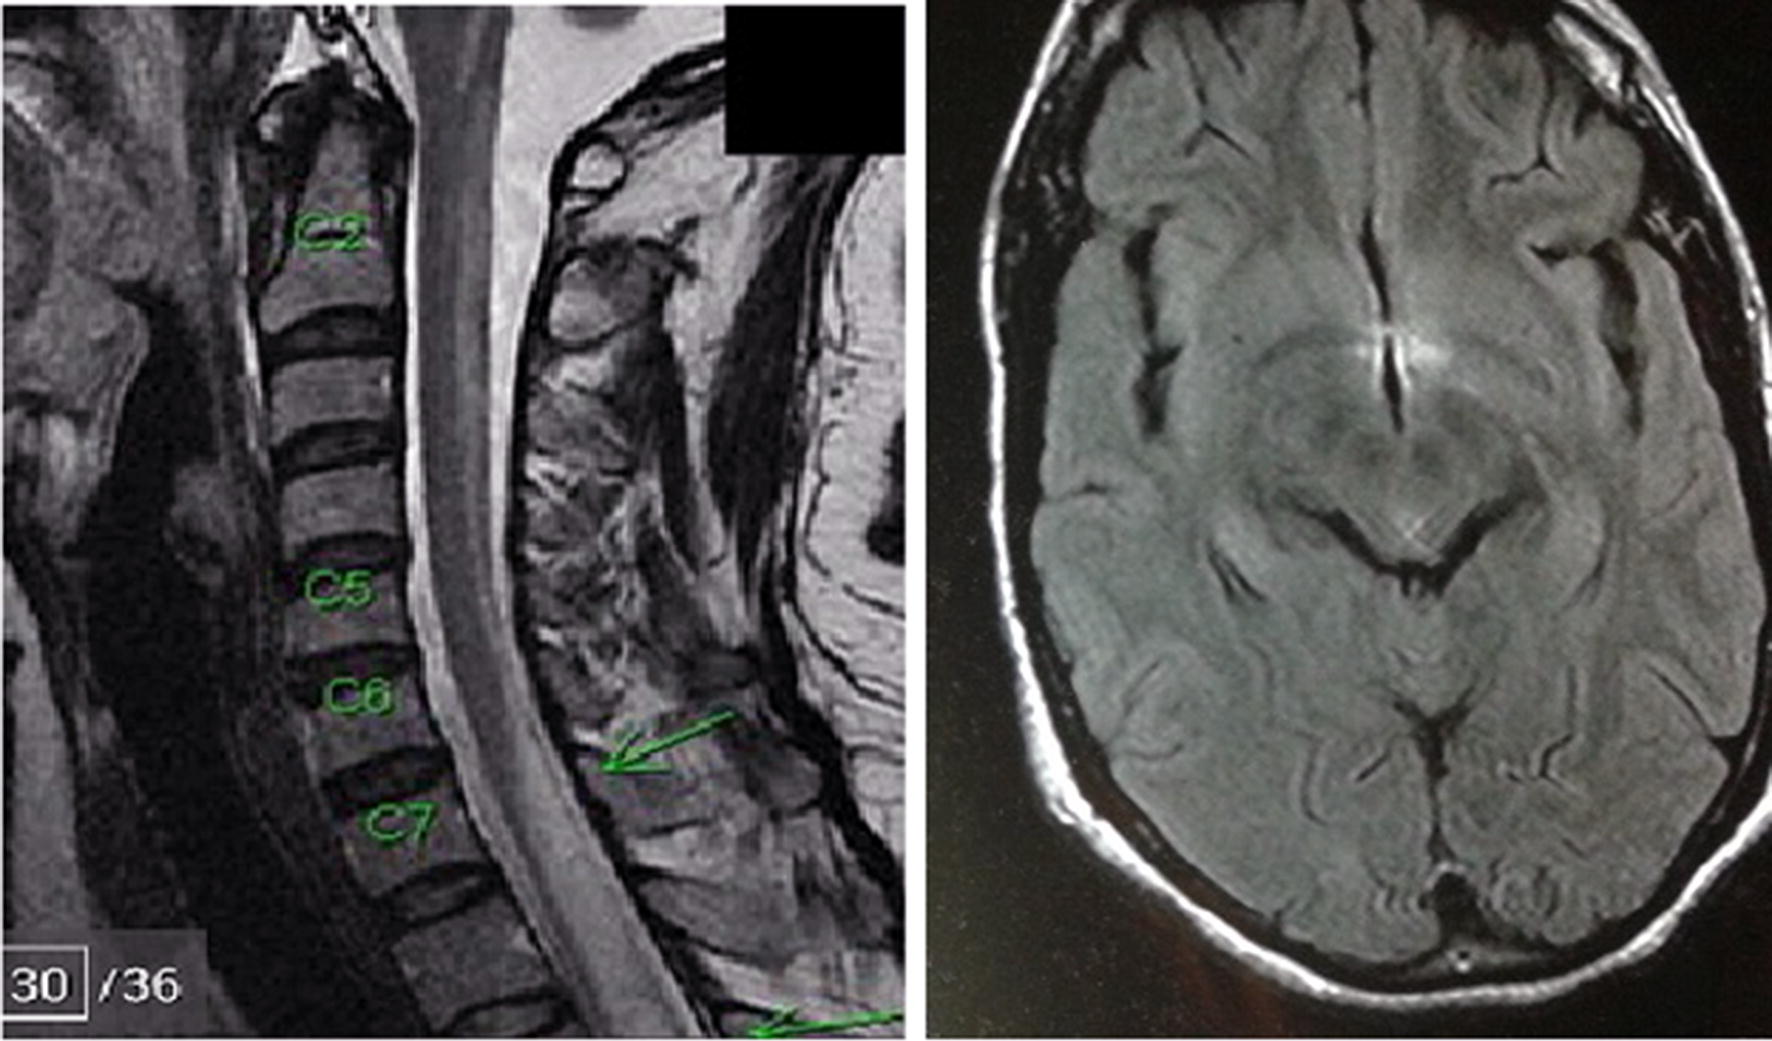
MRI of the brain showing hyper-intensity in the aquaporin4 area, and MRI of the cervical spine showing longitudinal hyper-intensities in more than 3 consecutive vertebrae in a patient with NMO presented with reduce vision both eyes and lower limbs weakness, over 2 month duration
